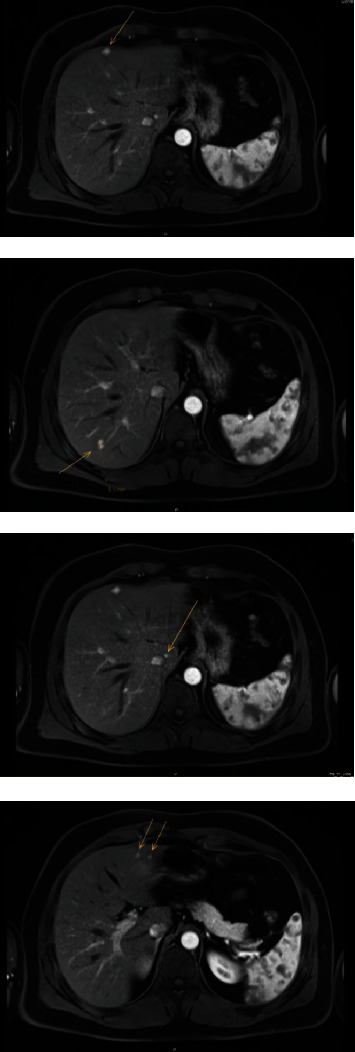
Liver MRI: demonstrates several liver lesions (segments 1, 3, 4, 7). These lesions were new compared to the liver MRI five years ago. One lesion in segment 3 and one lesion in segment 1 were new compared to the MRI scan of one month ago.
